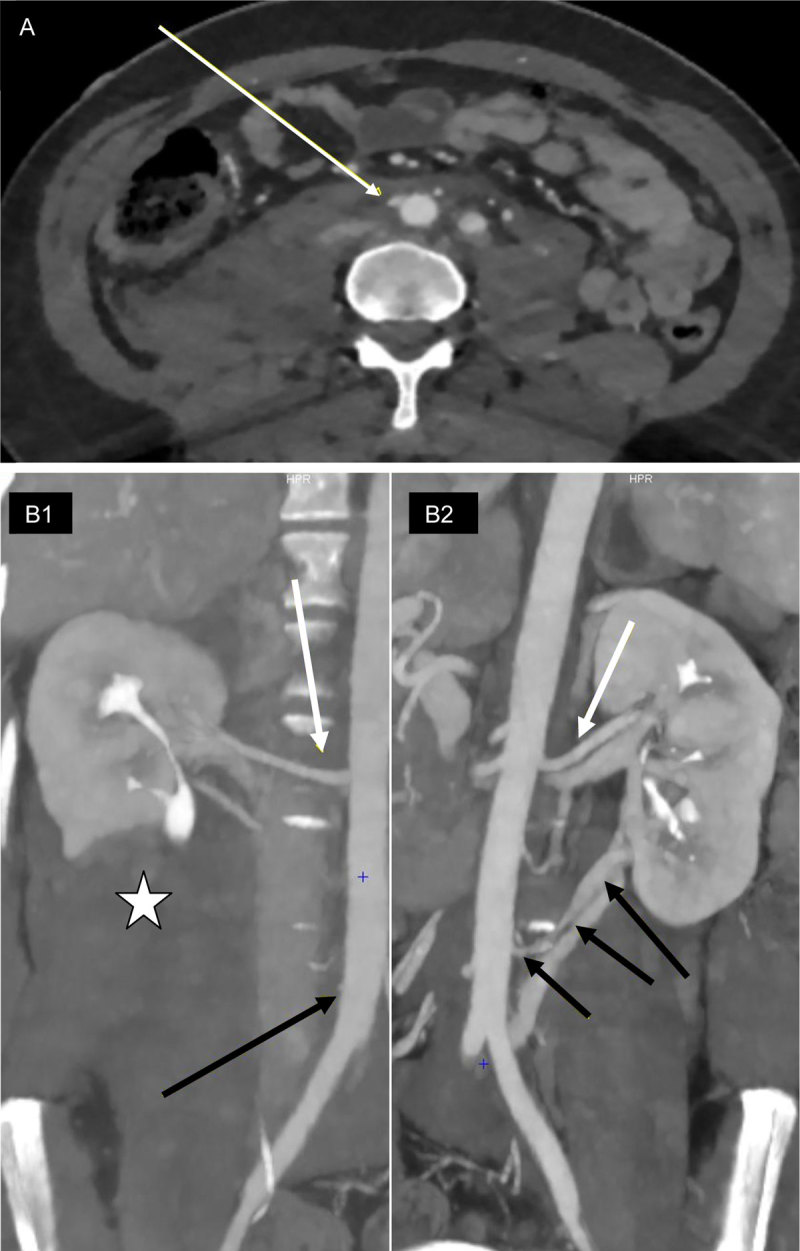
A: Enhanced axial CT of the aorta, with an ulcer-like contour (arrow) corresponding to ostial avulsion of the right polar inferior artery (RPIA). B: Oblique coronal views of the right (B1) and the left (B2) arterial vascularization of the kidneys, with two main renal arteries (white arrows), a left polar inferior artery (LPIA – B2, black arrows) and avulsion of the RPIA (B1, black arrow) associated to an infarction of the lower pole of the right kidney (white star).
